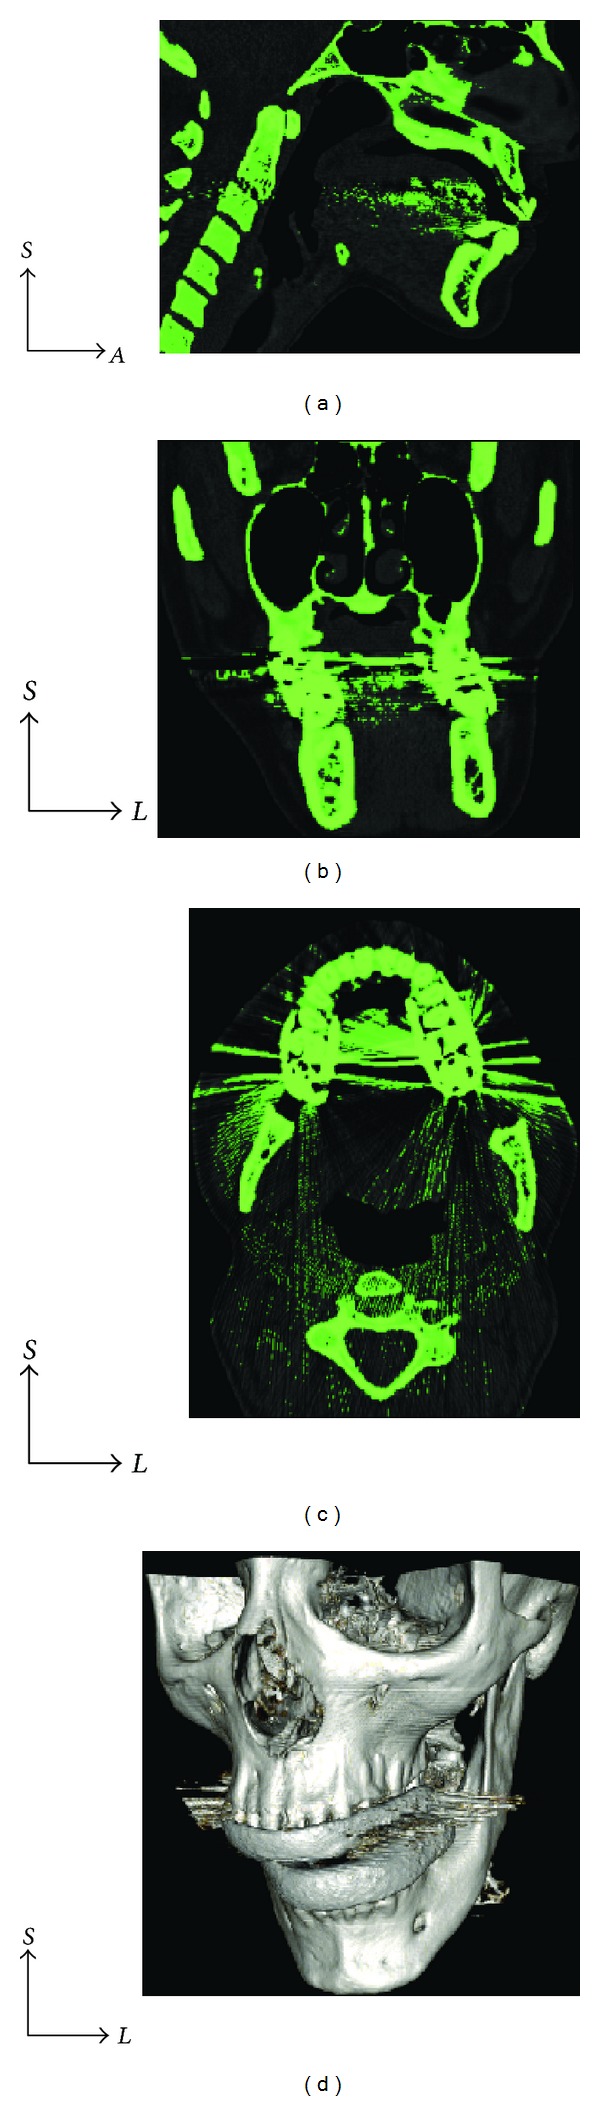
Sagittal (a), coronal (b), and axial (c) CT slices when imported into InVesalius. This software generates 3D reconstructions (d) from 2D DICOM CT or MR images.
